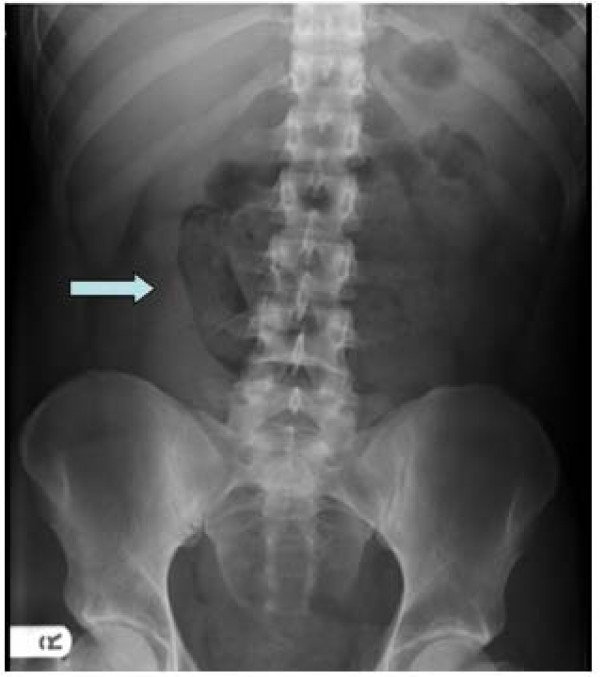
Plain abdominal x-ray showed a single loop of dilated small bowel (*arrow key*).
